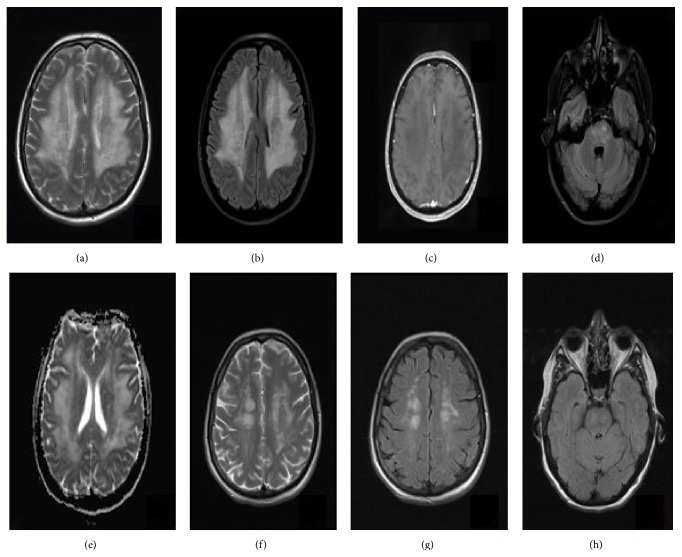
T2-weighted and FLAIR MRI revealed the presence of a high signal intensity mainly in periventricular and subcortical white matter of the bilateral cerebral hemispheres (a, b) and pons (d), without gadolinium enhancement on T1-weighted sequence (c). ADC maps showed increased signal intensity suggestive of vasogenic edema (e). The high signal intensity detected in the deep white matter of bilateral cerebral hemispheres was significantly reduced one year after the onset of symptoms under metronomic chemotherapy ((f) axial T2-sequence; ((g) and (h)) axial FLAIR-sequence).
